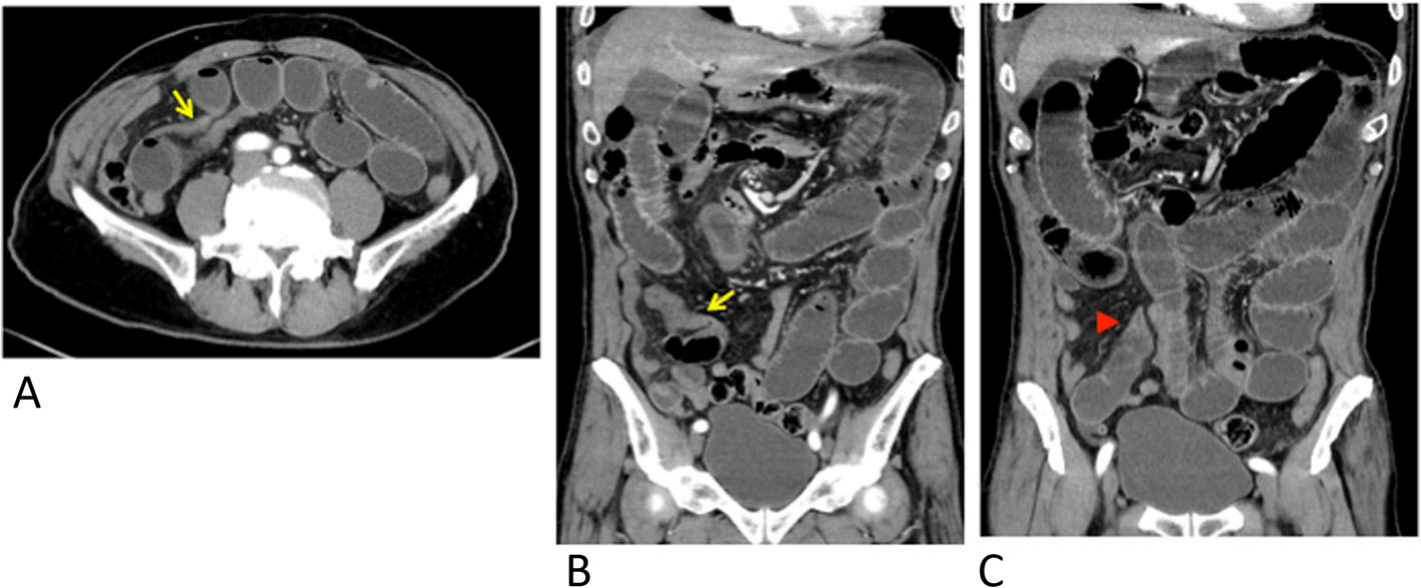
Enhanced abdominal computed tomography with arrows showing the border of the dilated segment of intestine (a, b) and an arrowhead indicating a fat notch (c)
